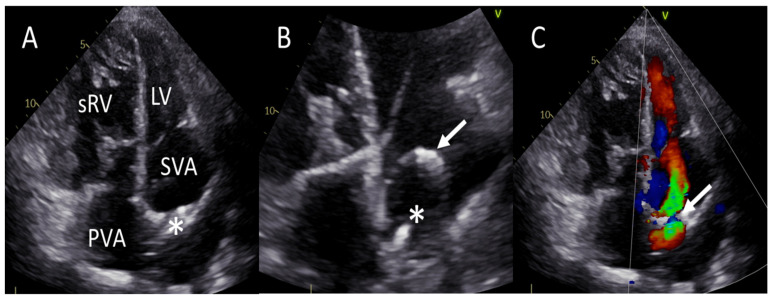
Baffle leak visualization in patient C. (A) Apical four chamber view shows the systemic venous return atrium (SVA), left ventricle (LV), the dilated and hypertrophied systemic right ventricle (sRV), and the pulmonary venous atrium (PVA). There is a suspicion of a baffle defect (asterisk (*) in (A)). (B) A small mobile structure suspicious of a vegetation (asterisk (*) in (B)) can be seen on the SVA side of the baffle defect. Note the atrial lead (arrow). (C) Color flow Doppler apical four chamber view demonstrates a PVA to SVA shunt over the baffle defect (arrow).
